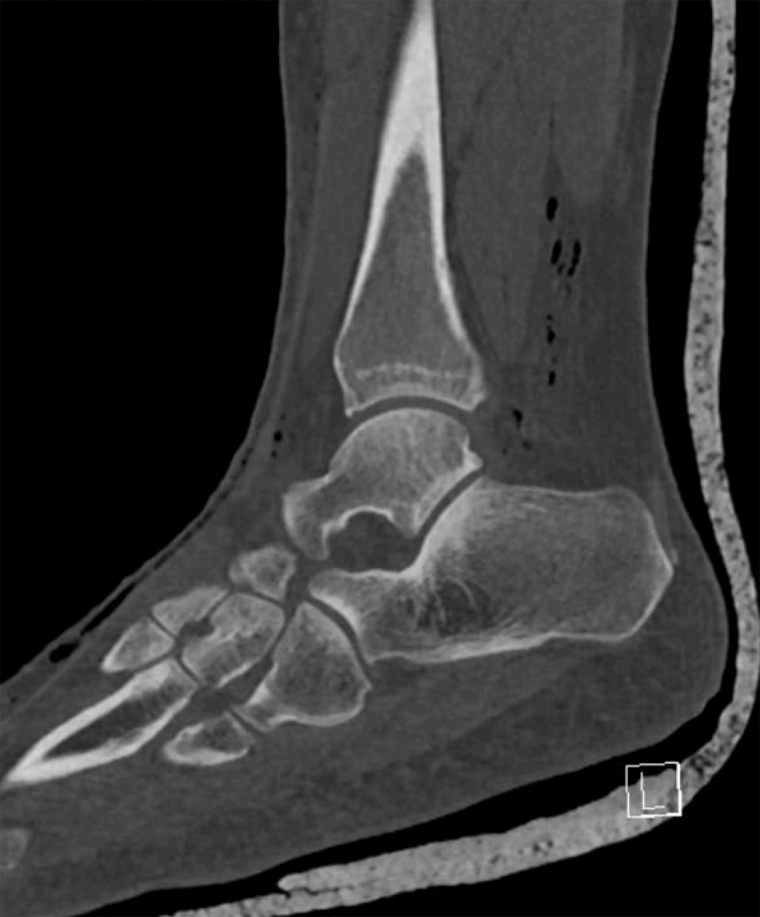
Postreduction computed tomography.
